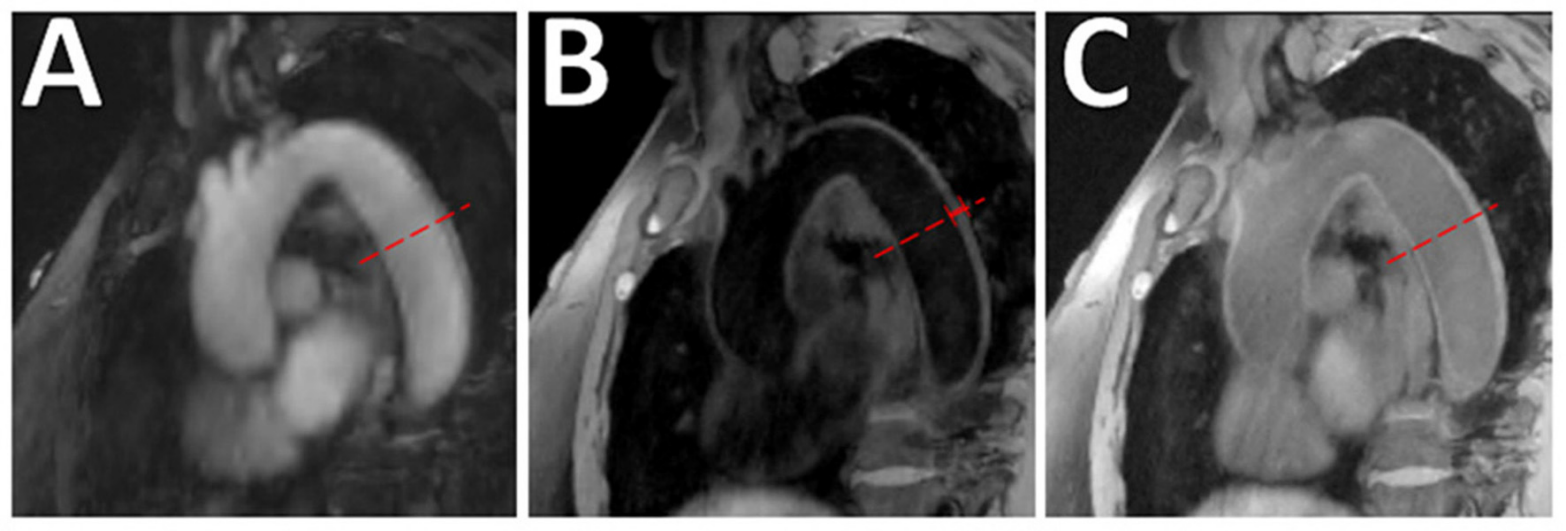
Aortic VW-MRI using the MR MultiTasking based 3D Multidimensional Assessment of Cardiovascular System (MT-MACS) Technique. (A) In a 71-year-old patient with aortic atherosclerosis, bright-blood lumenography, (B) dark-blood (vessel wall imaging), and (C) gray-blood (optimized to detect calcium/calcified nodules) images were acquired. In the thoracic aorta (dashed line), increased aortic wall thickness (4.491 mm) was measured and most conspicuous on the dark-blood (B) and gray-blood (C) images. [These images are re-printed with permission from Zhaoyang Fan, PhD from Magnetic Resonance in Medicine (112).]
